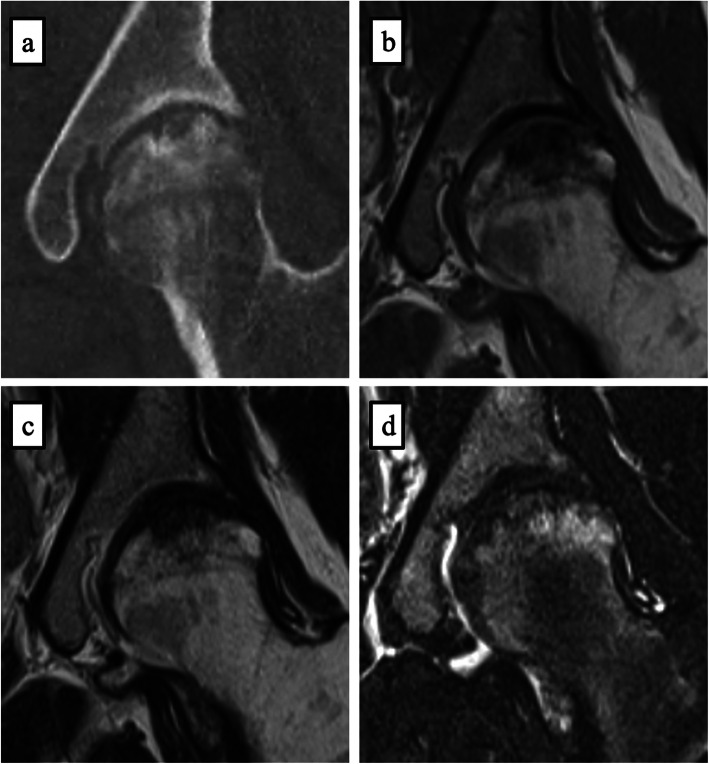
Plane CT and MRI (a: Coronal view of plane CT, b: T1 weighted image, c: T2 weighted image, d: Short TI inversion Recovery). Subcartilaginous cyst in the femoral head and edema were observed
